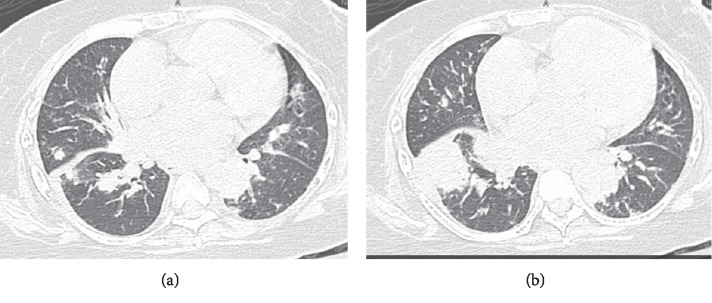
CT chest without contrast showed multifocal consolidations bilaterally with bibasilar predominance.
